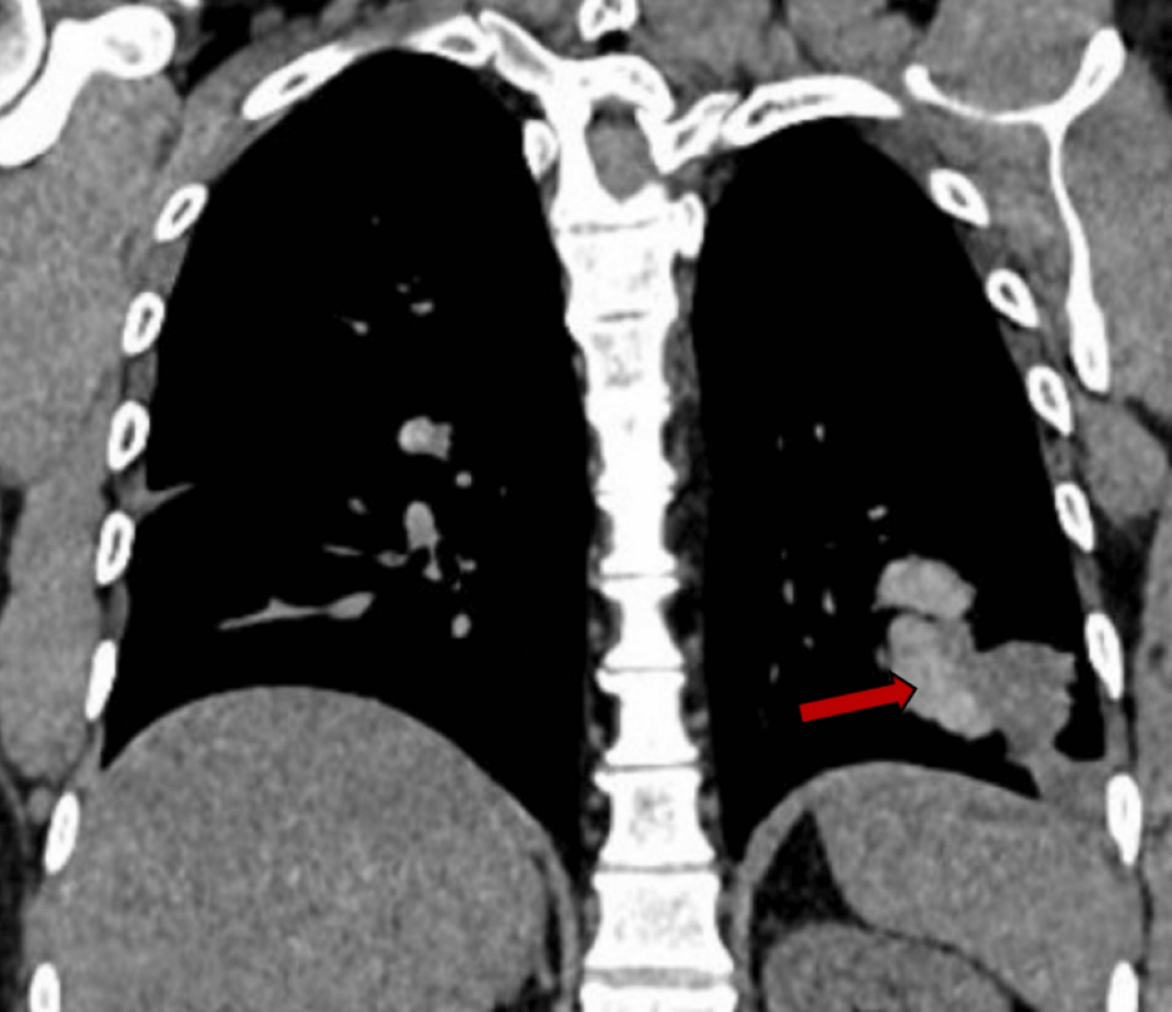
The CT of the chest (coronal, mediastinal window) demonstrates elongated, branching mucoid impaction within dilated bronchi of the left lower lobe. While not a classic “finger-in-glove” pattern, this represents a variant appearance of mucus-impacted bronchiectatic airways, typical of allergic bronchopulmonary aspergillosis, including a focus of high-attenuation mucus (red arrow).
